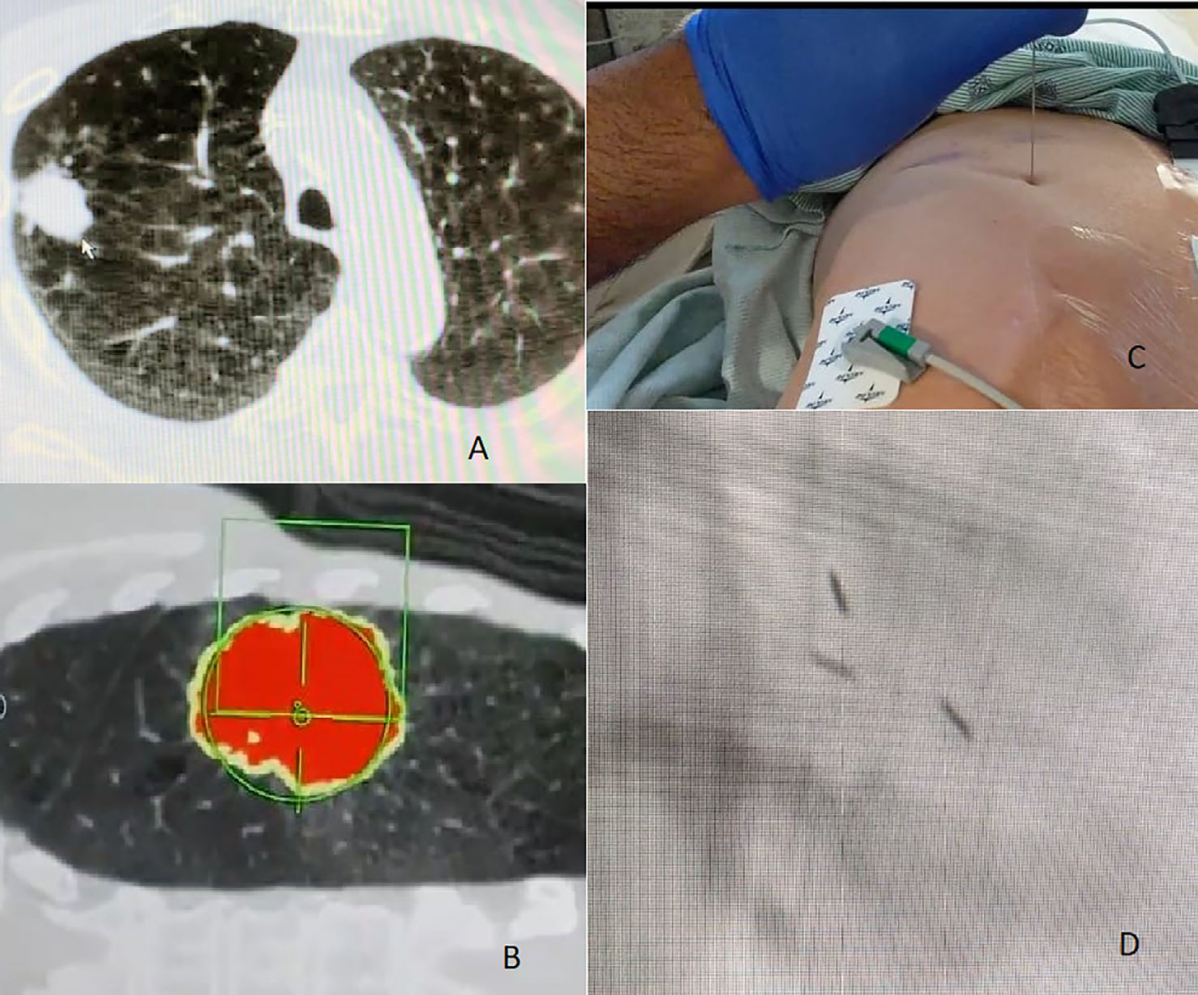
Example of an electronic navigational bronchoscopy (ENB) needle‐guided fiducial placement. A: Tumour seen on computed tomography. B: Biopsy planning with ENB needle. C: Fiducial placement through ENB needle trocar. D: Fiducials seen in the tumour on fluoroscopy
